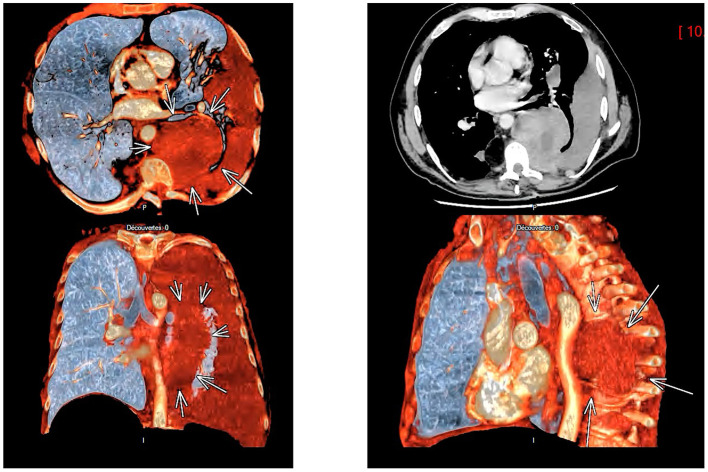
Multiple computed tomographic scan images of the chest with 3D reconstruction, revealing a large paravertebral soft tissue mass (arrows) compatible with extramedullary haematopoiesis and an adjacent left-sided haemothorax.
